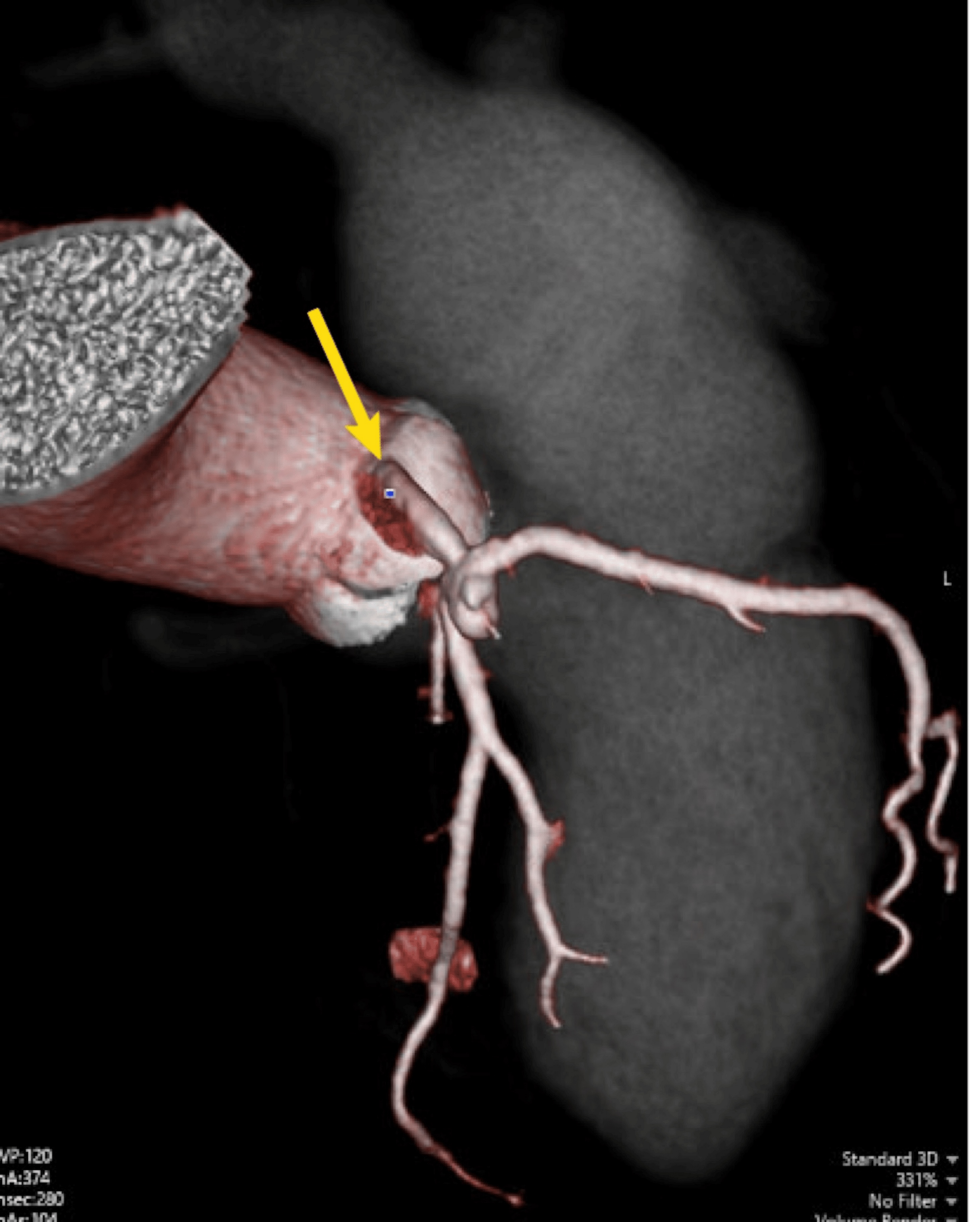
MDCT: volume rendered vessel reconstruction demonstrating all coronary arteries arising from the right coronary sinus. MDCT: multidetector CT
